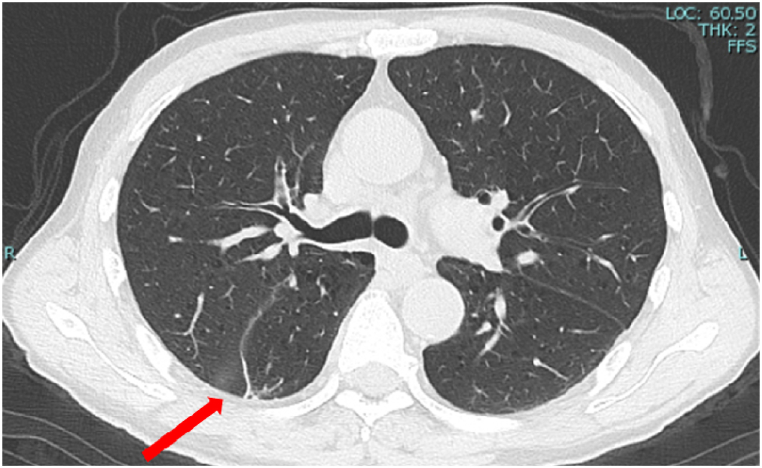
Chest CT on admission. A small scar-like nodular shadow can be seen on the pleural side of the right lower lobe (arrow).
